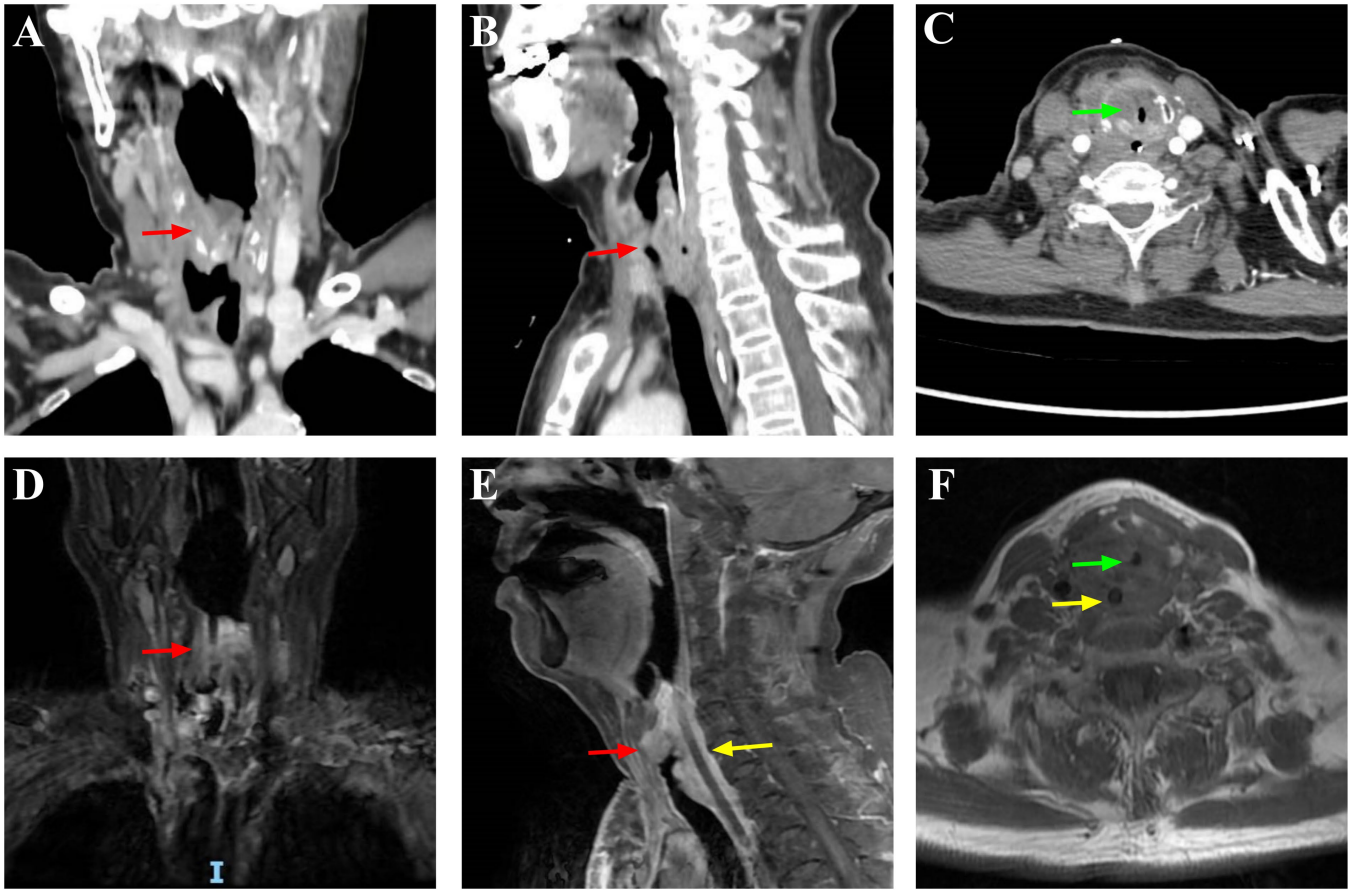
Preoperative neck imaging examination. CT: (A) Coronal view; (B) Sagittal view; (C) Axial view; MRI: (D) Coronal view; (E) Sagittal view; (F) Axial view. Red arrows in (A,B,D,E): Irregular mass in the right thyroid lobe with indistinct borders and unclear demarcation from the adjacent trachea and esophagus; Yellow arrows in (E,F): Tumor invasion into the esophagus; Green arrows in (C,F): Subglottic laryngeal stenosis and tracheal lumen invasion/narrowing.
